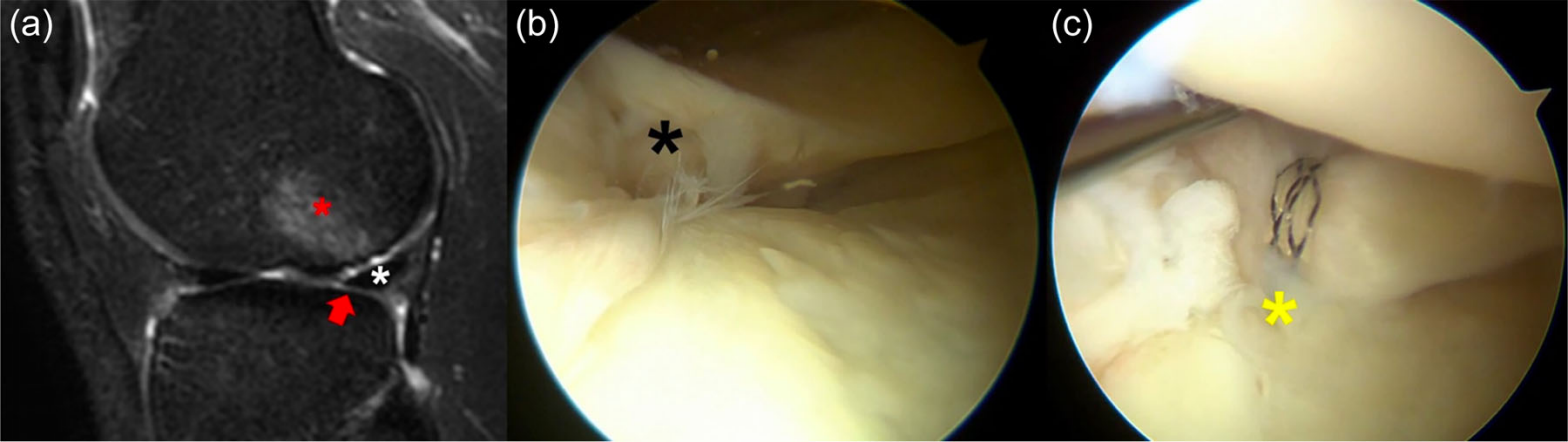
Left knee of a 29‐year‐old patient with a chronic ACL tear. The sagittal MRI of the lateral compartment shows the presence of subchondral bone oedema (red asterisk) and cartilage thinning (red arrow) at the lateral femoral condyle, with an intact lateral meniscus (white asterisk) (a). At the time of arthroscopy, the posterior root (black asterisk) was torn from the tibial insertion and floating (b). The root (yellow asterisk) was repaired with a transtibial tunnel (c). ACL, anterior cruciate ligament; MRI, magnetic resonance imaging.
